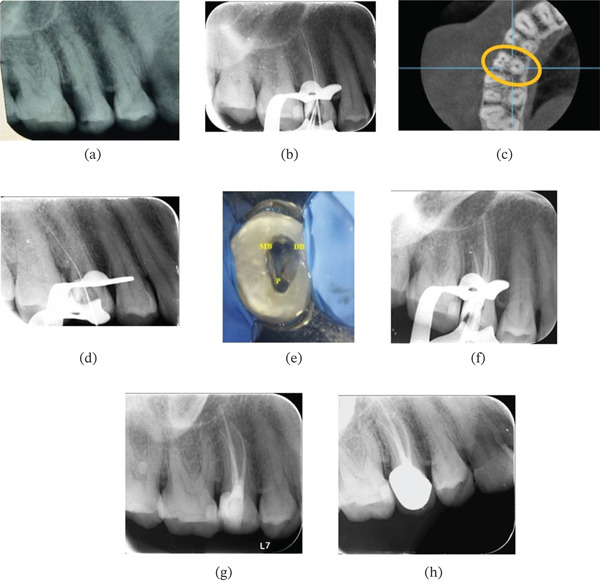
(a) Preoperative radiograph of 15; (b) working length radiograph—MB = 19 mm and P = 21 mm; (c) axial view of CBCT showing the presence of three distinct canals; (d) working length radiograph; DB = 19.5 mm; (e) T‐shaped access cavity showing three orifices under magnification; (f) master cone radiograph; (g) obturation and core build up radiograph; and (h) 1 year post treatment follow‐up radiograph with PFM full coverage crown.
